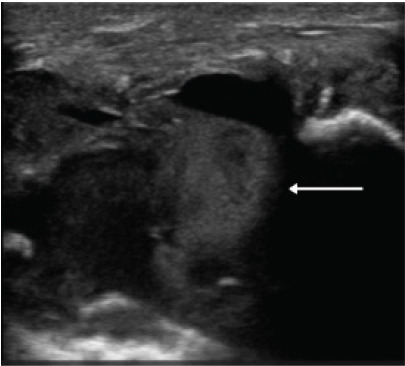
Ultrasound short-axis view of right subclavian artery pseudoaneurysm (arrow).
